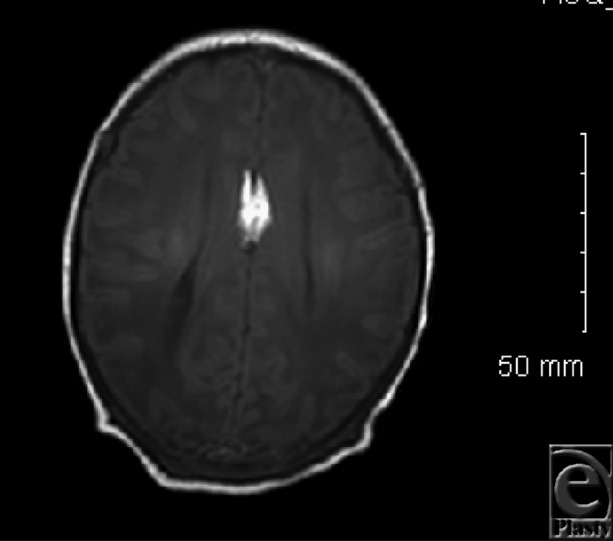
Magnetic resonance imaging demonstrating dysgenesis and lipoma of the corpus callosum.
